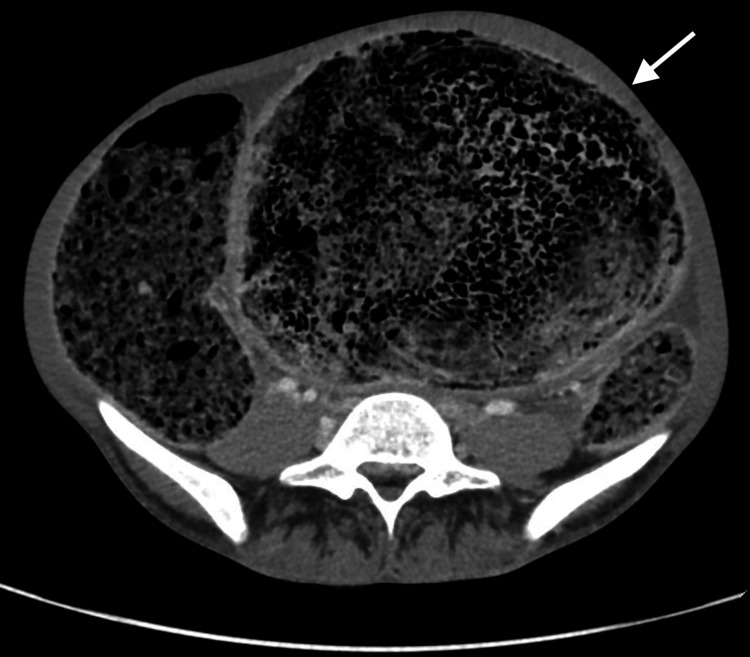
Axial CT scan with contrast displaying colon fully loaded with feces. The colon is 15 cm in width and 60-80 cm in length. This is an image of a huge dilated sigmoid colon that is loaded with feces.
